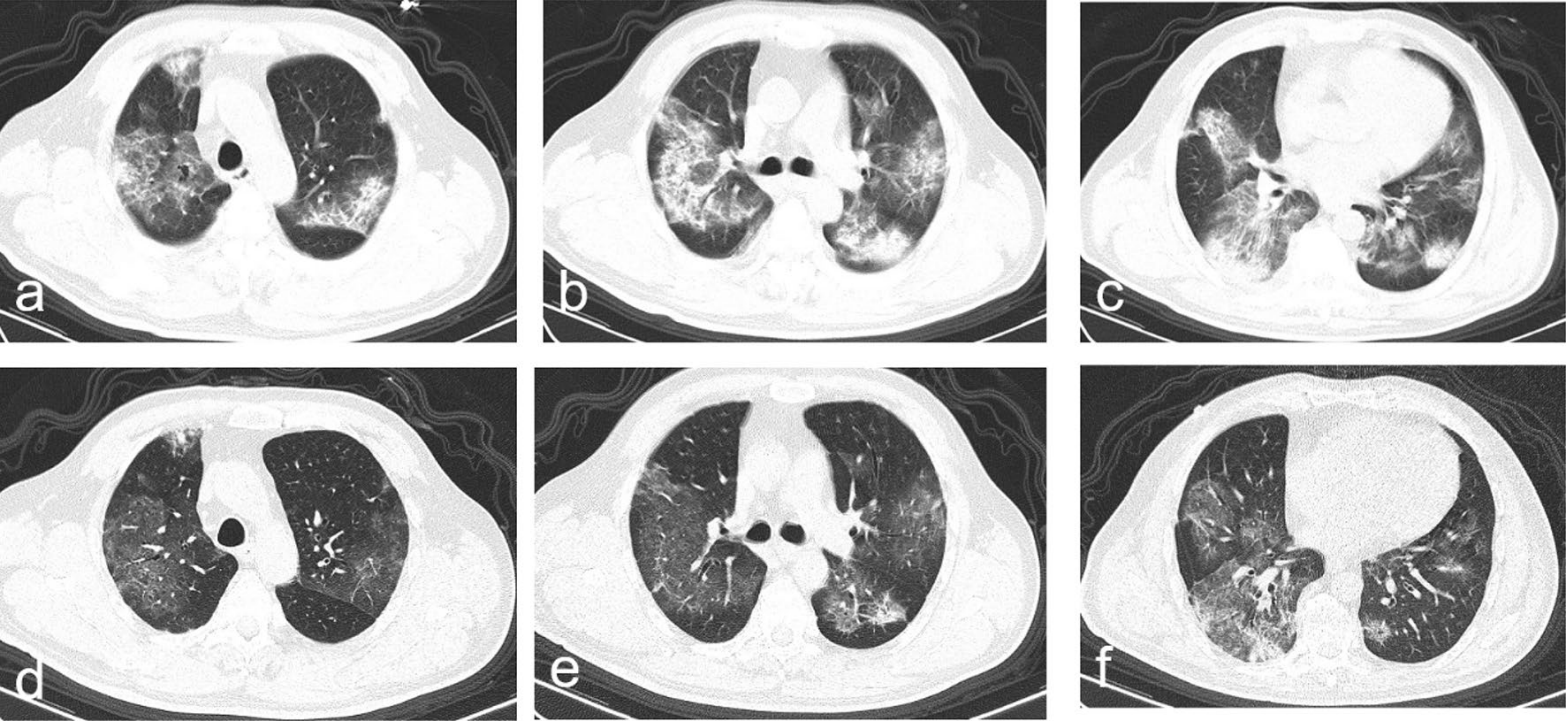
Transverse CT scans in a man with infected with SARS-CoV-2. Scan (a–c) obtained on 9th day from onset of symptoms shows bilateral, consolidation combined with ground glass opacities. Scan (d–f) obtained on 20th day shows previous consolidation being dissipated into ground-glass opacities. The patient was discharged from hospital 6 days after the final scan.
